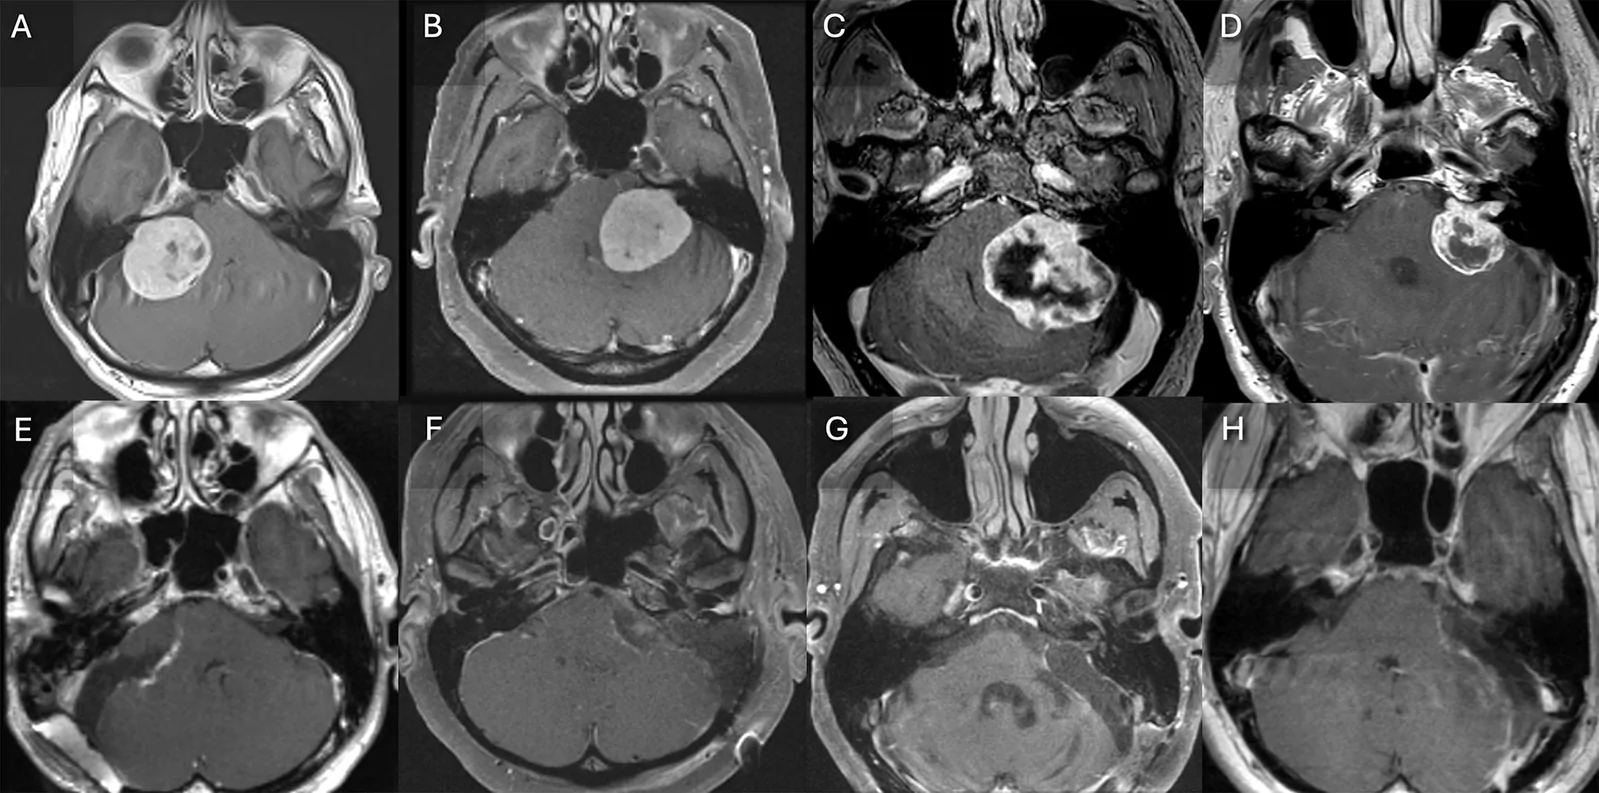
Representative collage of preoperative (A-D) and respective postoperative (E-H) MRI scans demonstrating patients with VS who underwent GTR and NTR with HB I-II outcomes. All patients shown in this figure had NTR except the patient in D and H who had GTR. Many patients who had NTR did not have radiographically visible nodular tumor (E-G)
